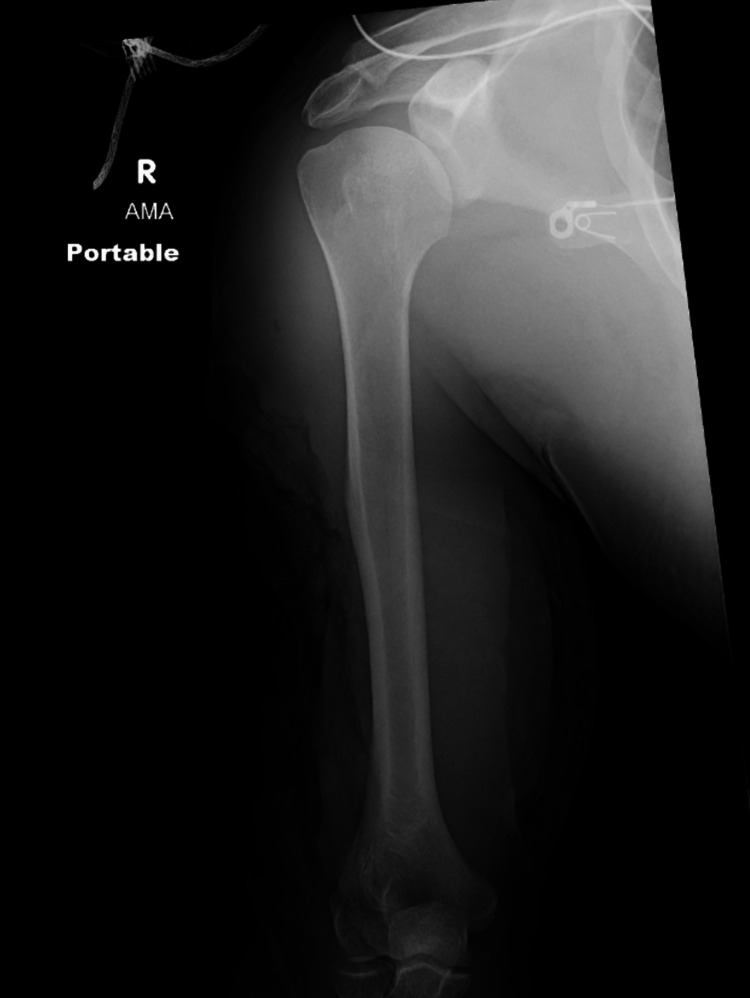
Radiography of the right arm demonstrating extensive soft tissue defects with overlapping soft tissue gas, equivocal for early osteomyelitis.
